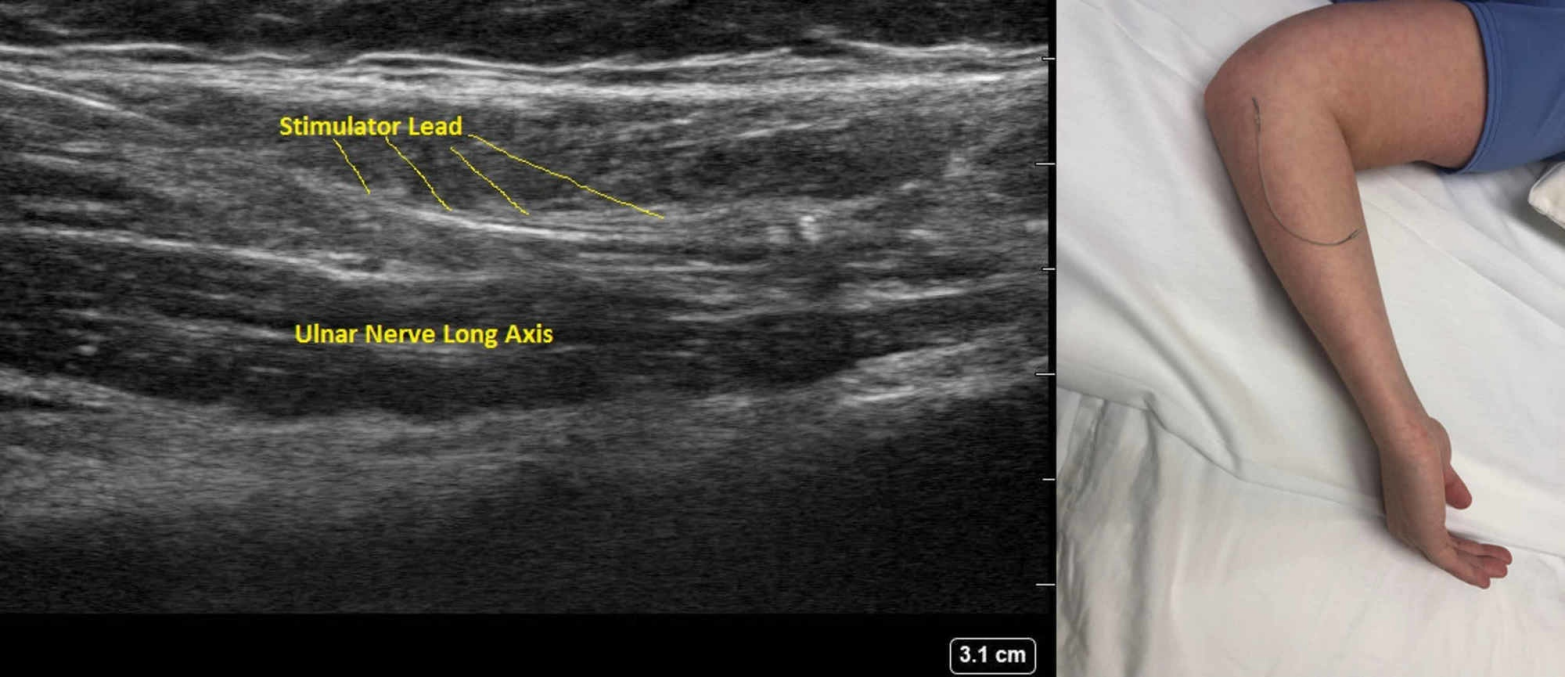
Administration of percutaneous peripheral nerve stimulation Left side: longitudinal ultrasound image of the stimulator lead being implanted along the trajectory of the left ulnar nerve Right Side: percutaneous peripheral nerve stimulator placed over a volunteer’s forearm skin, showcasing the trajectory of the lead implanted in the patient
